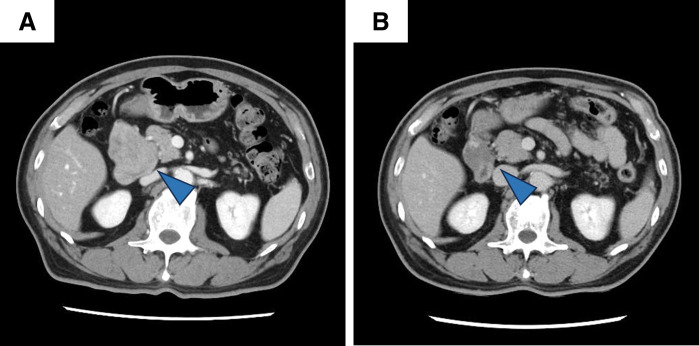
Enhanced CT findings before and after neoadjuvant chemotherapy. (A) A 55-mm tumor was located in the duodenal bulb. (B) After imatinib therapy, the tumor size decreased to 27 mm. No evidence of pancreatic invasion was observed. Blue arrowheads indicate the tumor.
